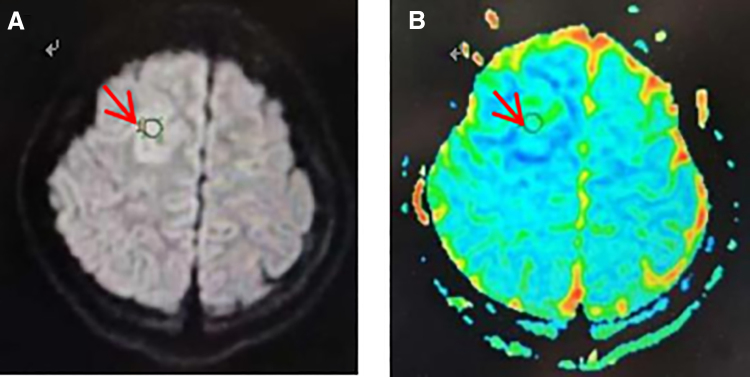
(A) The transverse sequence of the brain DWI; (B) The ADC perfusion sequence. ADC = apparent diffusion coefficient, DWI = diffusion-weighted imaging, MRI = magnetic resonance imaging.
